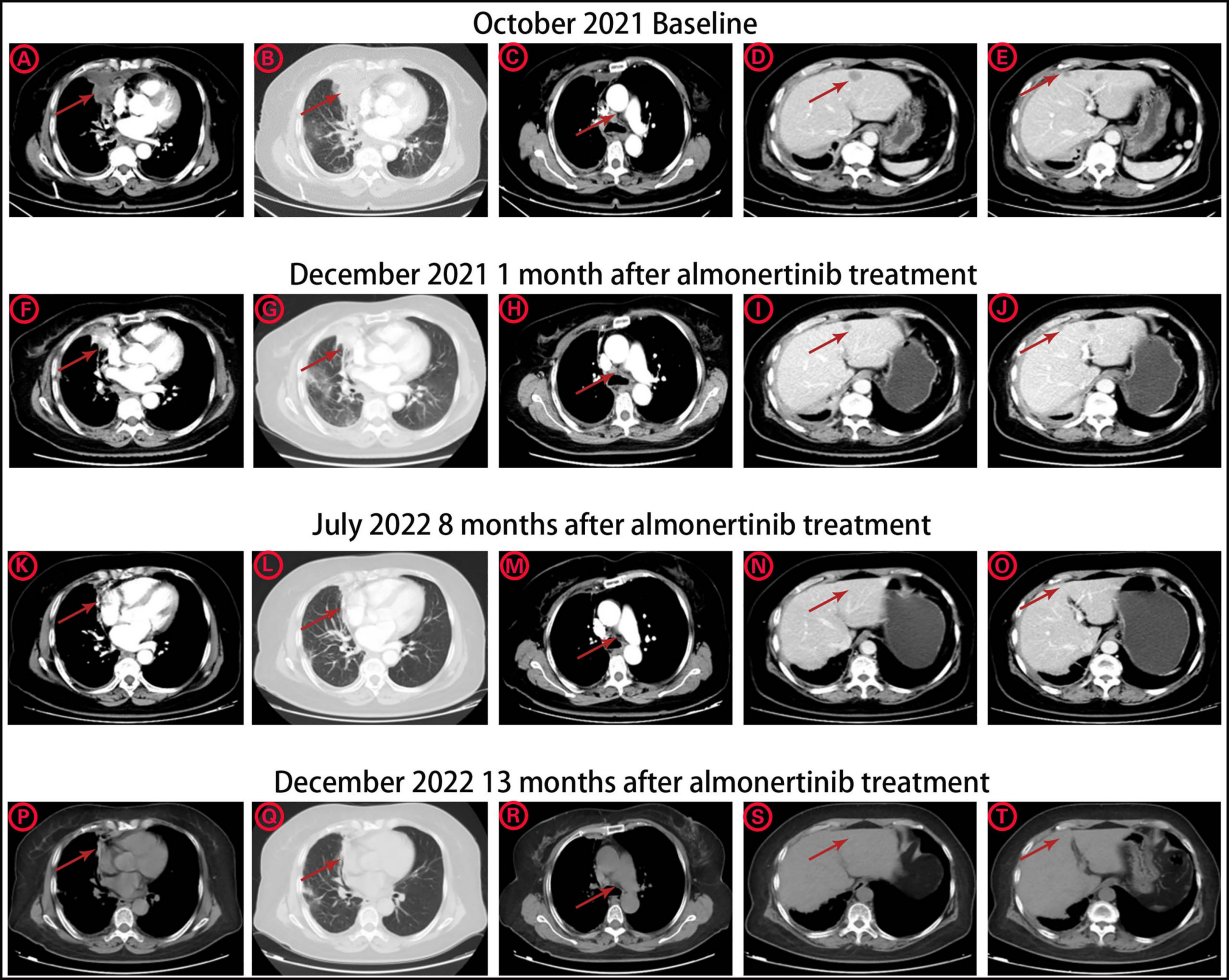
The CT image changes of pulmonary primary tumor site, mediastinal lymph node metastasis, and liver metastases (A-E) before Almonertinib treatment in October 2021. (F-J) 1 month after Almonertinib treatment in December 2021. (K-O) 8 months after Almonertinib treatment in July 2022. (P-T) 13 months after Almonertinib treatment in December 2022.
